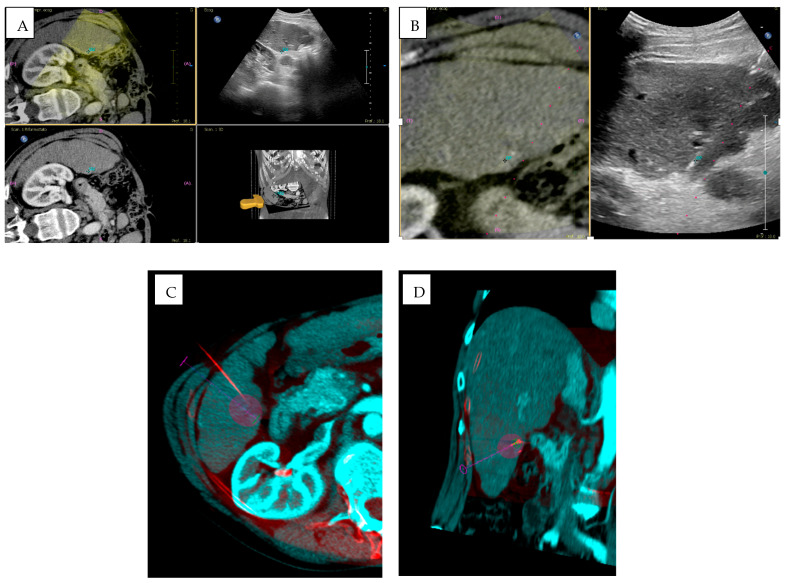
A 72-year-old man with HCC treated with MWTA under fusion imaging guidance. (A) Pre-procedural planning using fusion of CT and ultrasound images to identify the target lesion. (B) Intra-procedural monitoring during insertion of the MWTA antenna into the target lesion. (C,D) Prediction of the ablation zone using XperCT software (EPIQ PercuNav, Philips fusion system) (purple circles).
